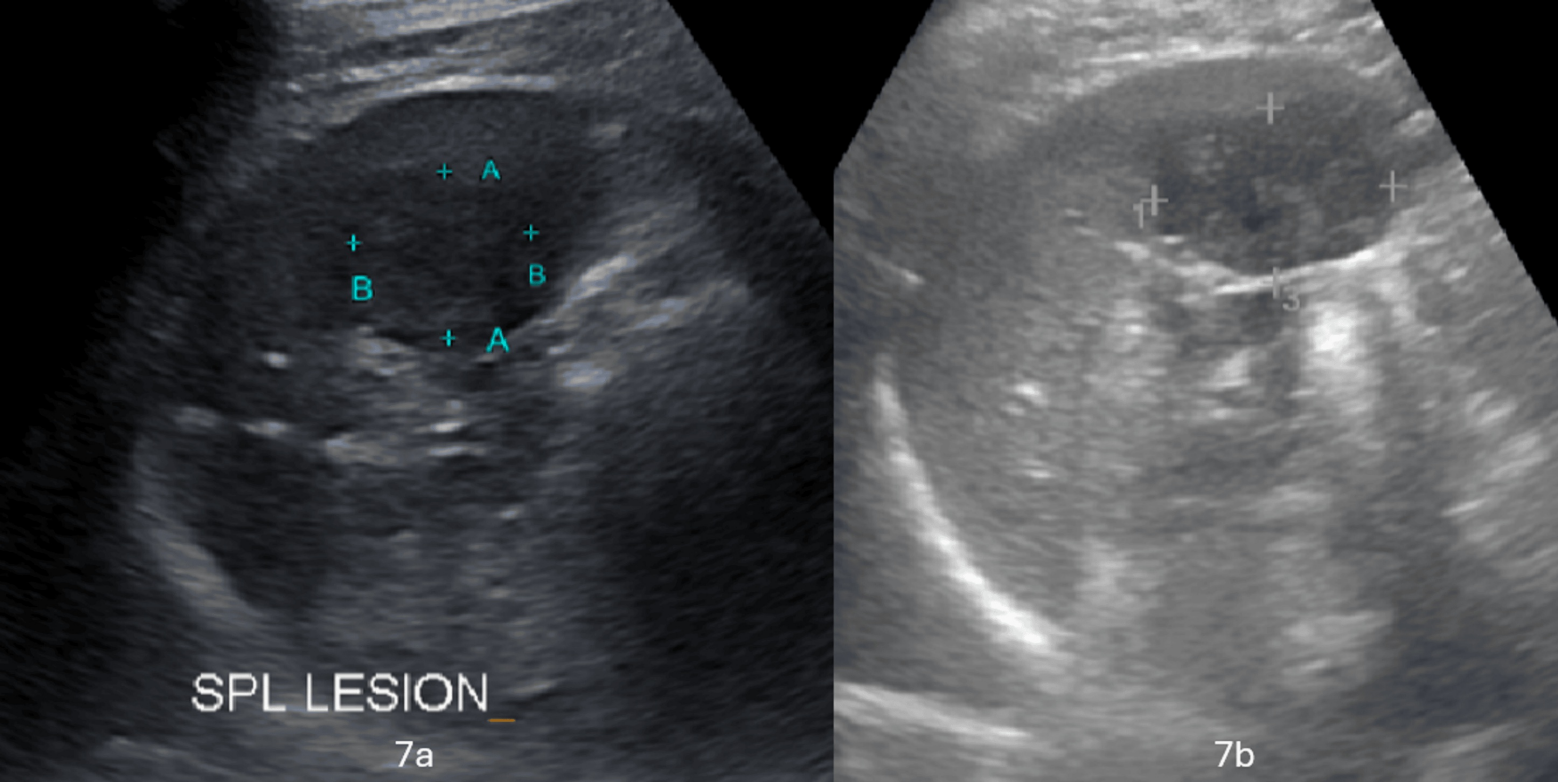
Follow-up ultrasound Ten-month (7a) and two-year (7b) follow-up images showing no significant interval change, measuring 4.5 × 4.3 cm and 4.3 × 4.0 cm, respectively.
